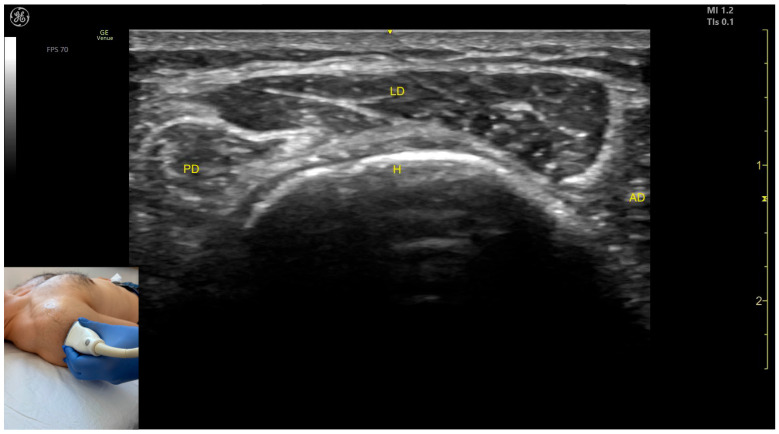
Ultrasound anatomy of the lateral deltoid (LD) with key landmarks: PD—posterior deltoid; LD—lateral deltoid; AD—anterior deltoid; and H—humerus.
